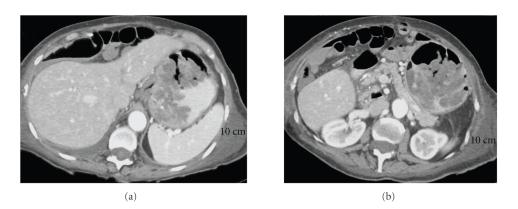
(a) Axial CT through the gastric cardia demonstrates large lobular folds forming a mass-like configuration. (b) Axial CT through the gastric body shows a distended stomach with multiple lobular mass-like folds.
